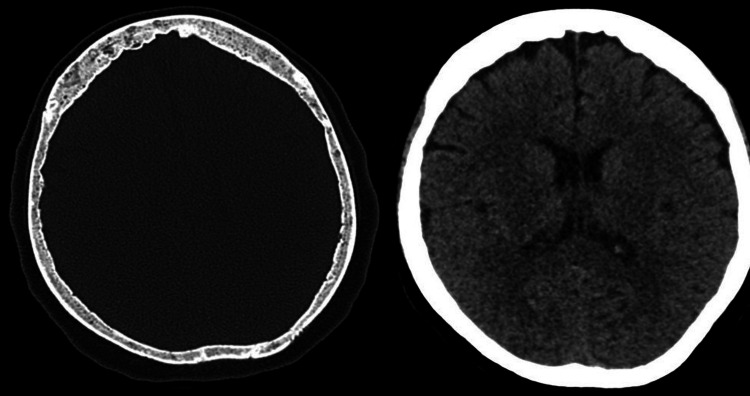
CT head without contrast showing normal gray-white matter differentiation of both cerebral hemispheres, and multiple small well-defined lytic lesions of the skull vault CT: computed tomography
